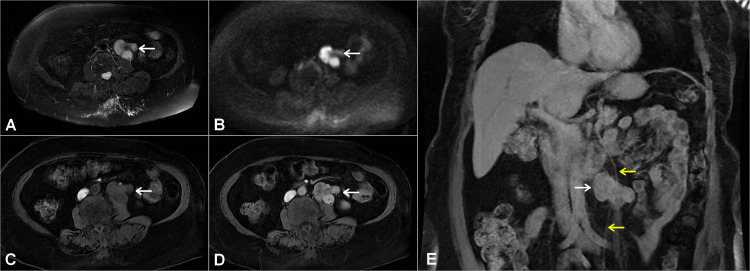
Abdominal MRI image showing a retroperitoneal macroscopic-fat free soft tissue mass that was completely separated from the ipsilateral psoas muscle (white arrows) (A) Axial T2 and (B) diffusion-weighted MRI images showing a mass with high signal intensity. (C) Axial T1-weighted MRI image showing a mass with low signal intensity and (D) heterogeneous gadolinium enhancement. (E) Coronal T1-weighted MRI image showing the left ovarian vein (yellow arrows) running throughout the mass.
